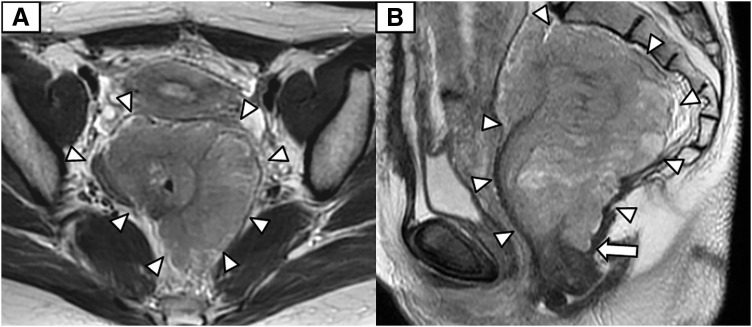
Pelvic MRI. T2-weighted images show a well-circumscribed, low-signal-intensity mass circumferentially surrounding the lower rectum. (A) Axial view shows the lesion in broad contact with the uterus. (B) Sagittal view shows the lesion extending dorsally and caudally toward the anal verge (white arrow). White arrowheads indicate the tumor margins.
